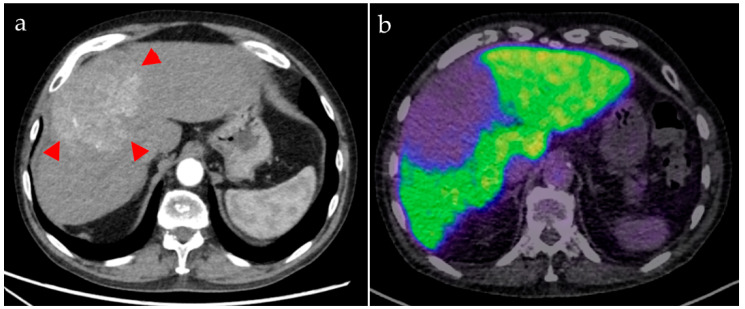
Patient A. The contrast-enhanced CT (a, arterial phase) shows the hypervascularized HCC ((a), arrowheads), involving both liver lobes. [^68^Ga]Ga-BP-IDA PET/CT (b), acquired 10 min after tracer injection, shows low uptake in the tumor (SUV_max_ 4.9) compared with healthy liver tissue (SUV_max_ 18.2).
